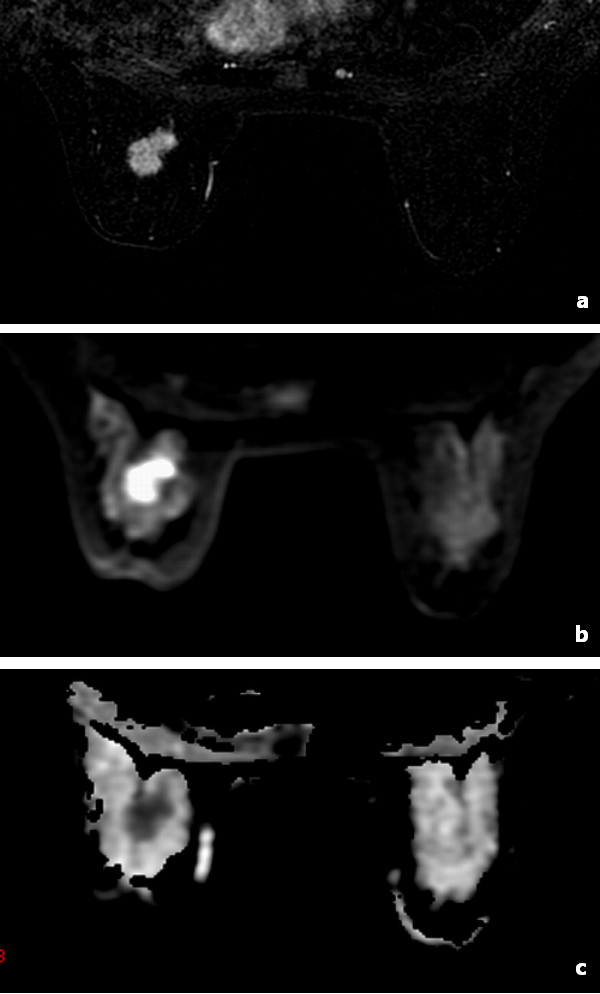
MR images of a 49-year-old woman with invasive ductal carcinoma. MR images of a 49-year-old woman with invasive ductal carcinoma on (a) early contrast-enhanced subtraction image, (b) gray-level DW image, and (c) ADC map.
